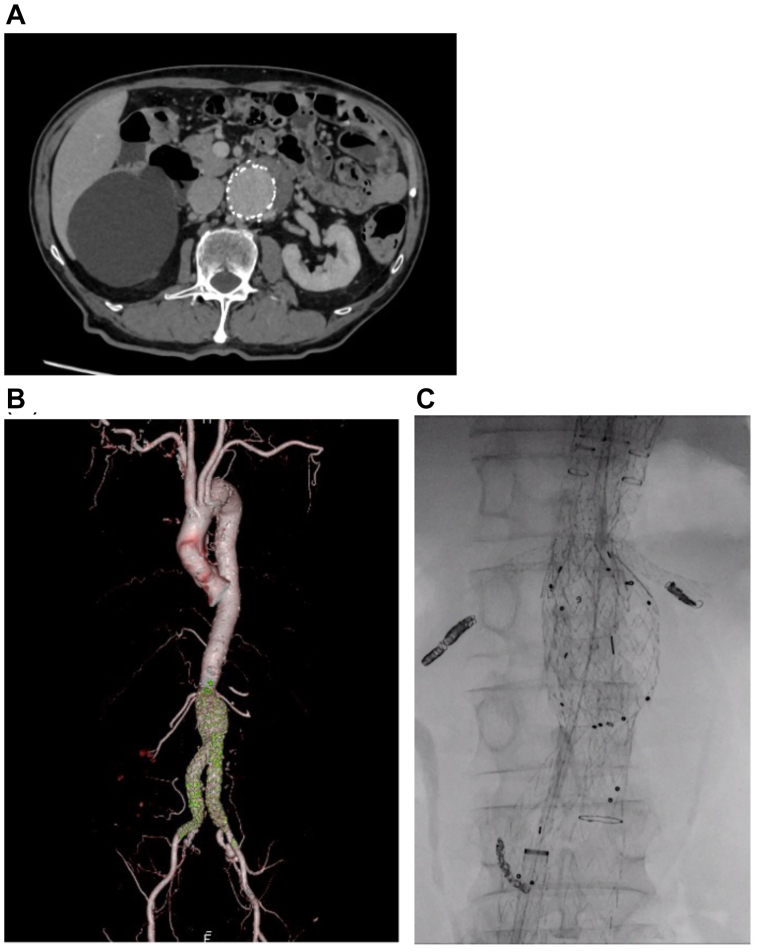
Pre- and intraoperative images. A, Computed tomography (CT) image of preoperative type IA endoleak. Preoperative CT angiography demonstrating type IA endoleak. B, Three-dimensional (3D) reconstruction of preoperative CT angiogram. Previous infrarenal endovascular aneurysm repair with suprarenal fixation. C, Intraoperative image after distal bifurcated component (DBC) full deployment showing congested operative field.
